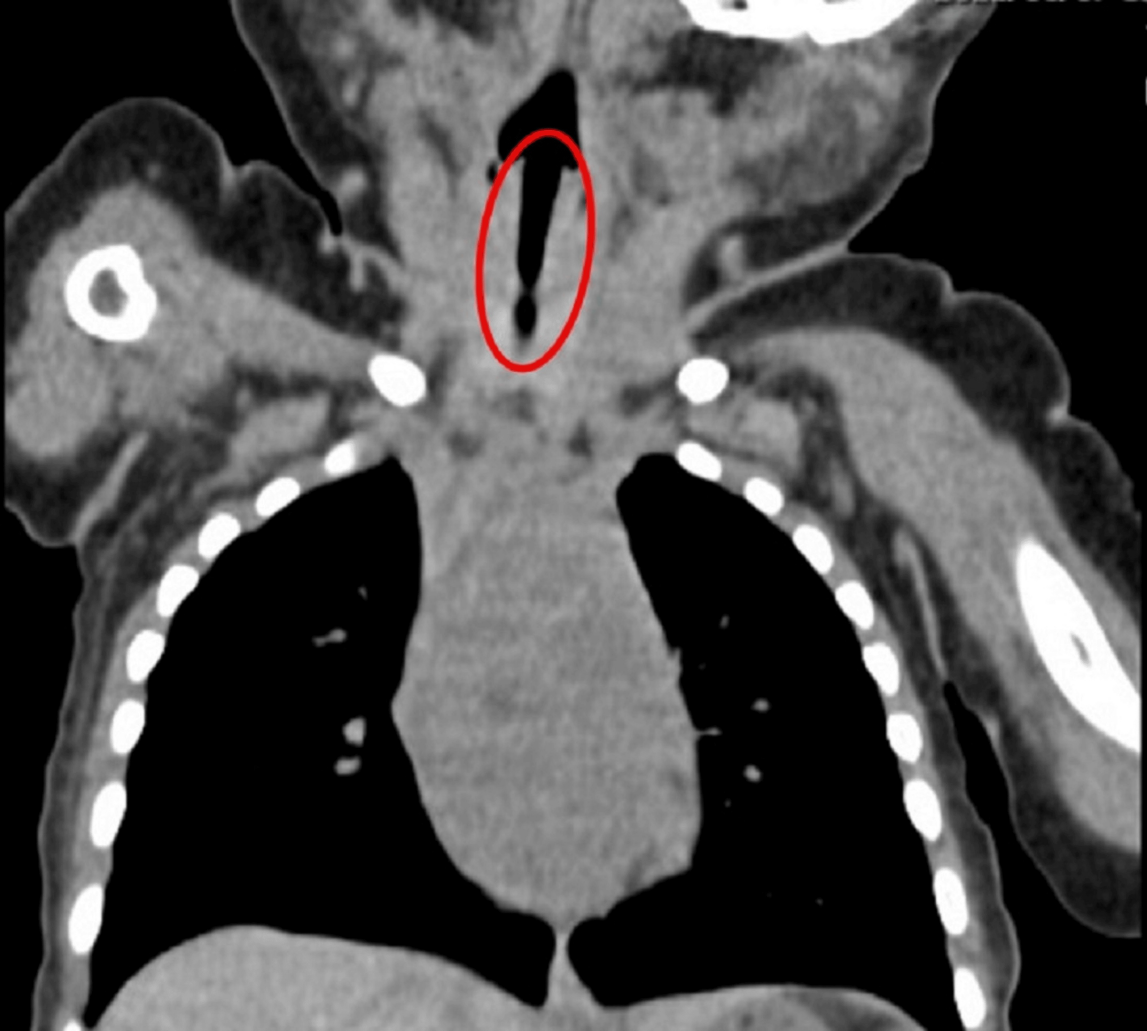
Findings on cervical CT No deformity and stenosis involving tracheal cartilage was observed (red circle).
